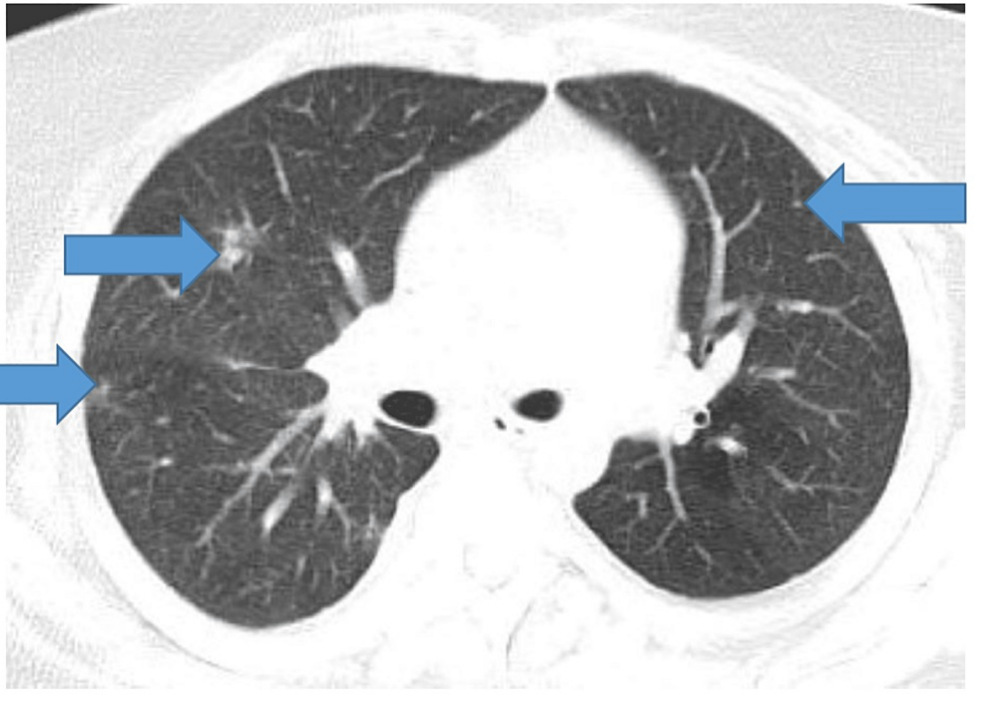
CT chest with arrows pointing to bilateral pulmonary nodules
